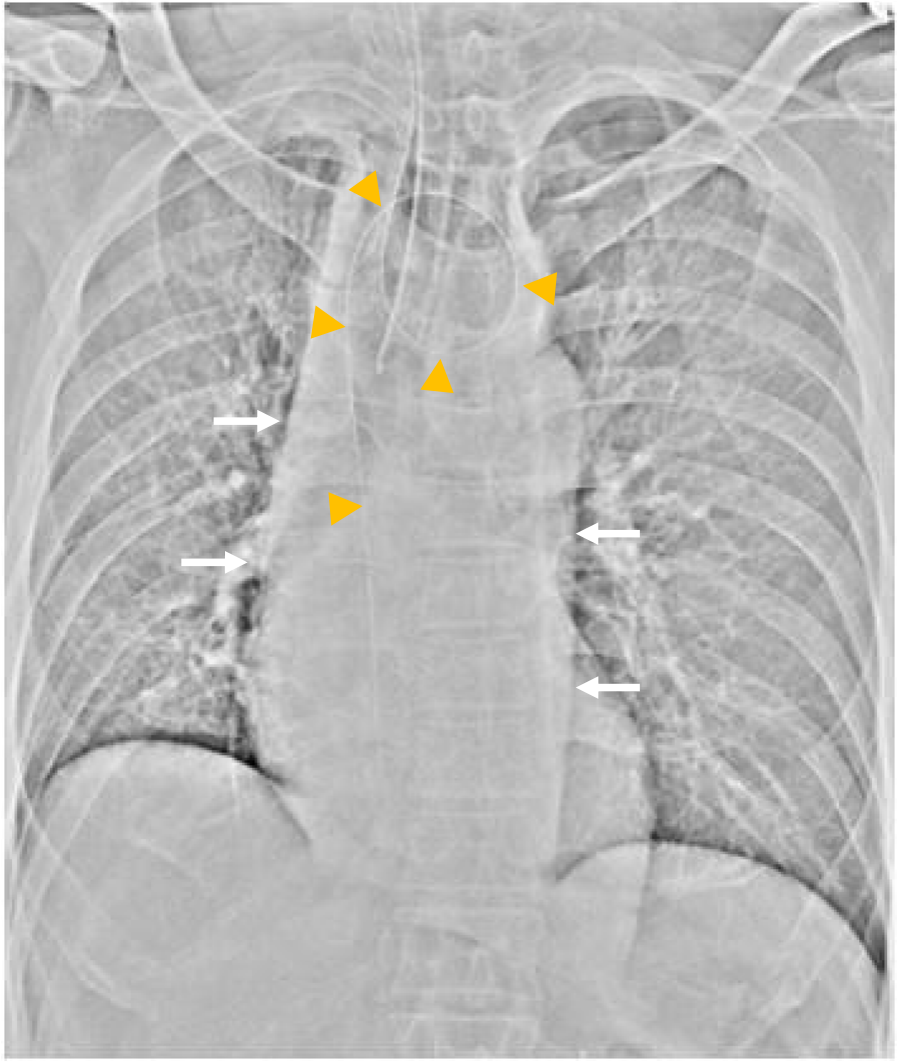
Chest X-ray image demonstrating enlargement of the mediastinum (white arrows). The stomach tube had formed a loop in the dilated esophagus (yellow arrowheads)
